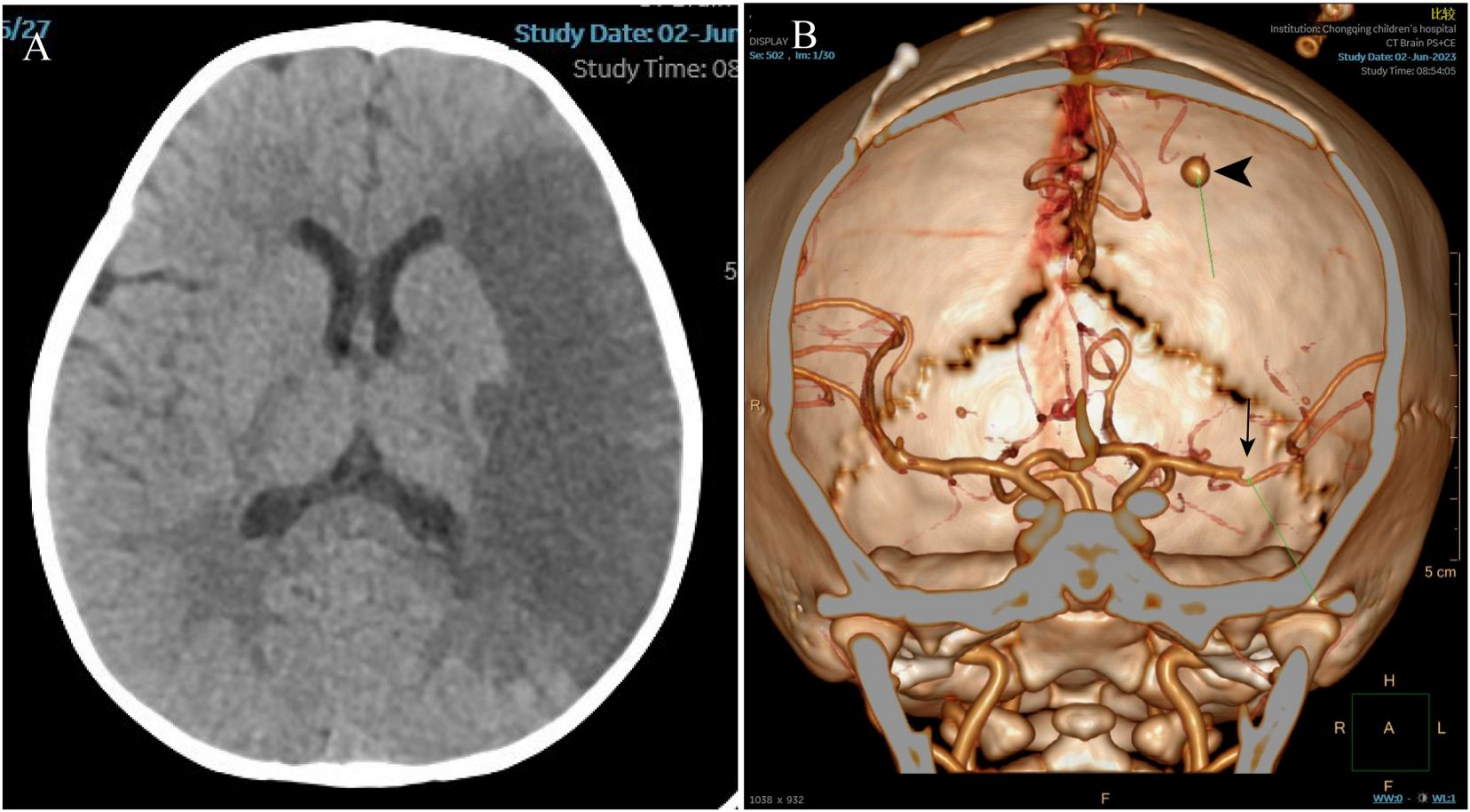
In‐department imaging examination at admission: (A) CT scan presented embolic cerebral infarction of left temporal lobe. (B) CTA scan presented upper branch occlusion of middle cerebral artery (MCA) (black arrow) and infectious intracranial aneurysms (IIA) located in distal artery region (DAR) of ipsilateral MCA (black arrow head). CTA, computed tomography angiography.
